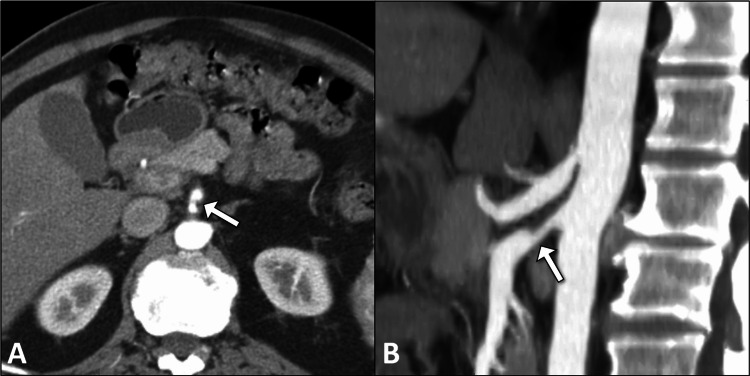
Axial and sagittal contrast-enhanced CT images showing SMA narrowing Axial (A) and sagittal (B) contrast-enhanced abdominal CT images demonstrate focal narrowing of the SMA (arrows), suggestive of chronic mesenteric ischemia. No evidence of thrombosis or aneurysmal dilation is seen. CT: computed tomography; SMA: superior mesenteric artery.
